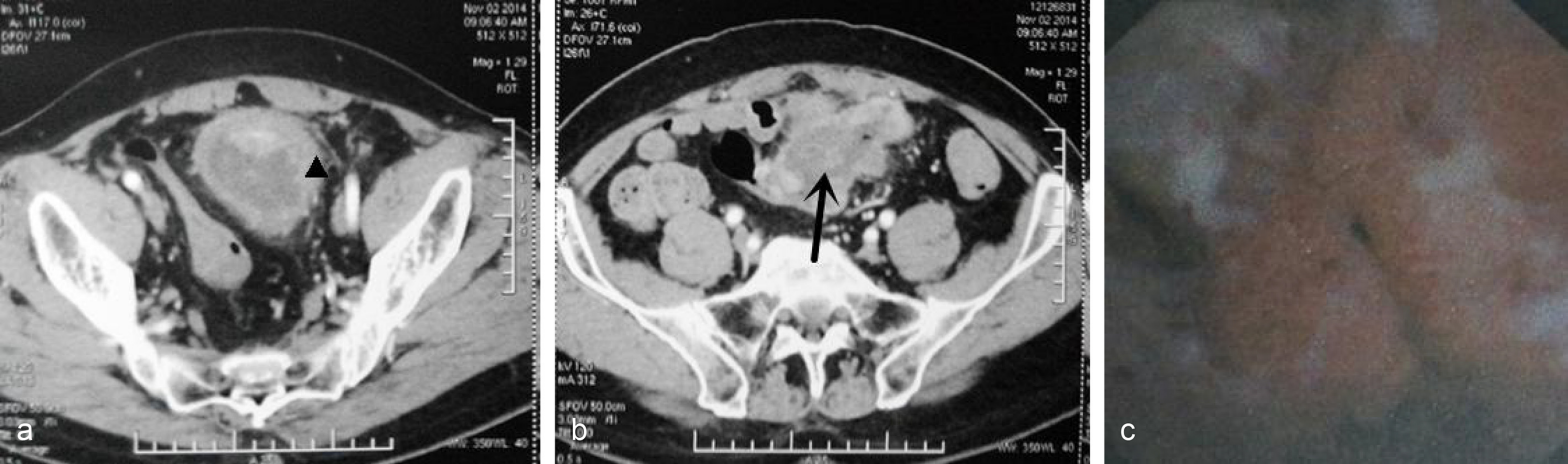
(a) CT scan showed the bladder wall to be irregularly thickened and the serosa was coarsened. (b) Protrusion of soft tissue shadows was visible on the left anterior part of the bladder (size, 4.6 × 6.8 cm). The mean CT value was 30 Hounsfield units, and the enhanced CT value was 67 Hounsfield units. The bladder wall was not smooth but it had multiple enhanced nodules. (c) A substantial mass, which appeared to be a tumour (size, 4 × 4 cm), had several floccules adhering to it.
